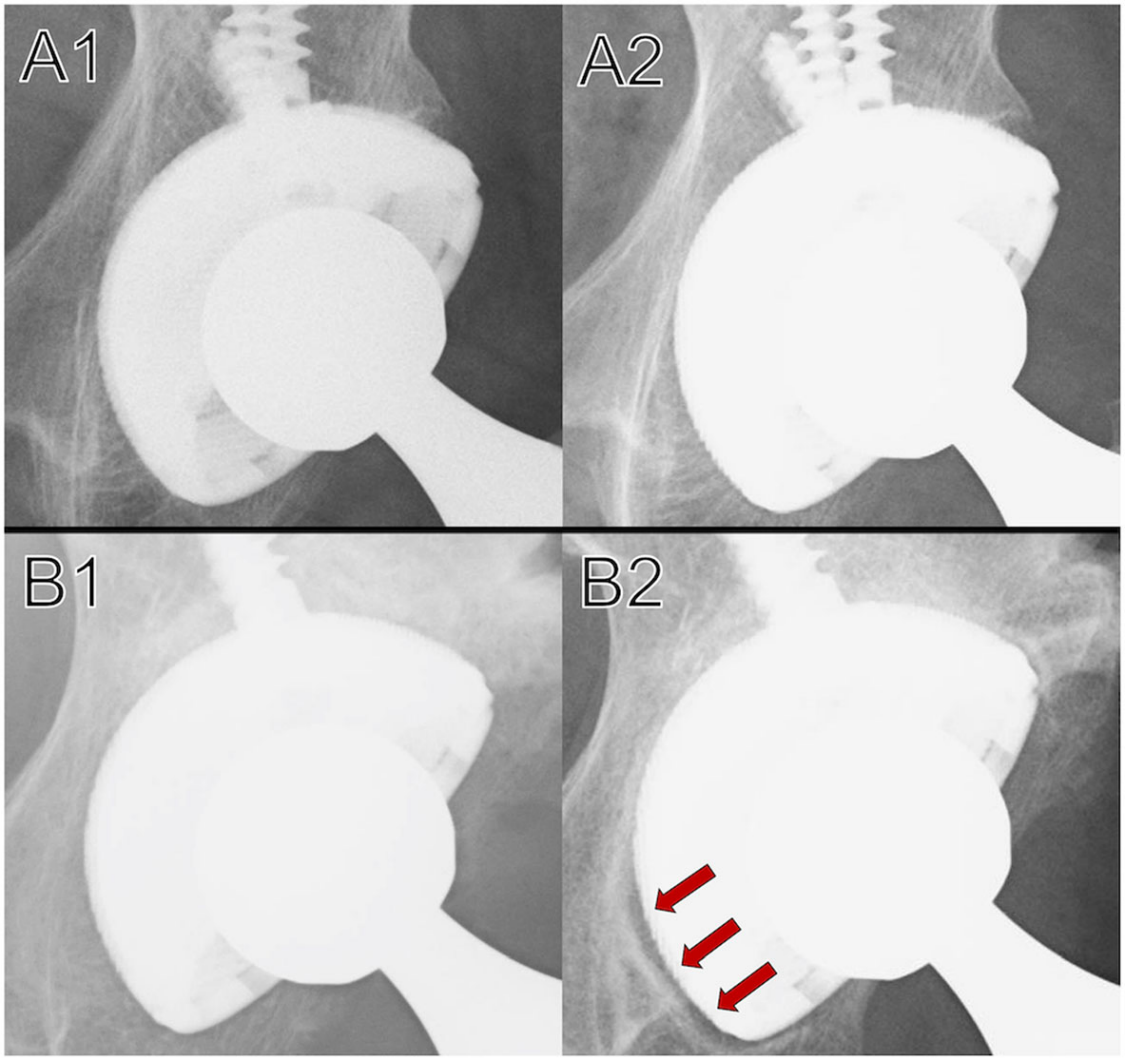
Radiograph images in which radiolucent lines did not appear and where they did appear. A1, B1: There is no initial gap on the anteroposterior radiograph immediately after surgery. A2: There is no radiolucent line around the cup 2 years after surgery. B2: Two years after the operation, radiolucent lines are observed in DeLee and Charnley Zone 3 (red arrows)
